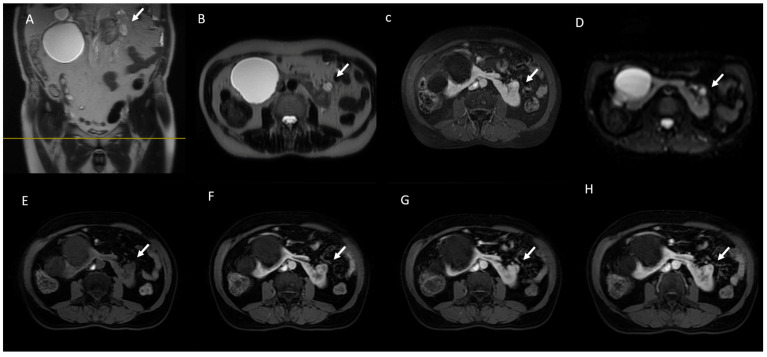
MRI assessment of low-grade clear cell RCC (arrows). (A) Coronal and (B) axial T2-W images show a small renal mass with a hyperintense signal, without FS in T2-W FS (C) and restricted diffusion (D). (E) Axial FS gradient T1W image shows hypointense lesion that after using a contrast agent, has heterogeneous enhancement ((F): corticomedullary phase; (G,H): nephrogenic phase).
